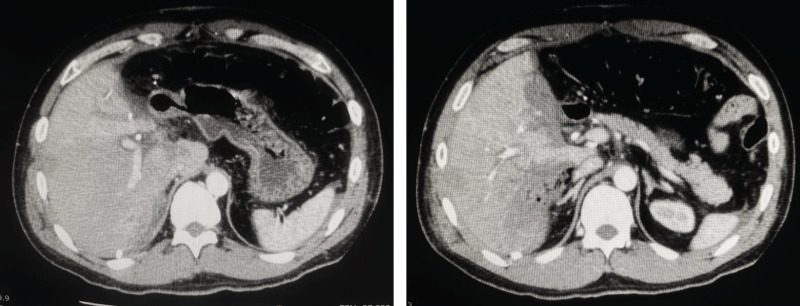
Contrast-enhanced CT images obtained on the day after the reoperation. The hepatic hemangioma was not detected.
